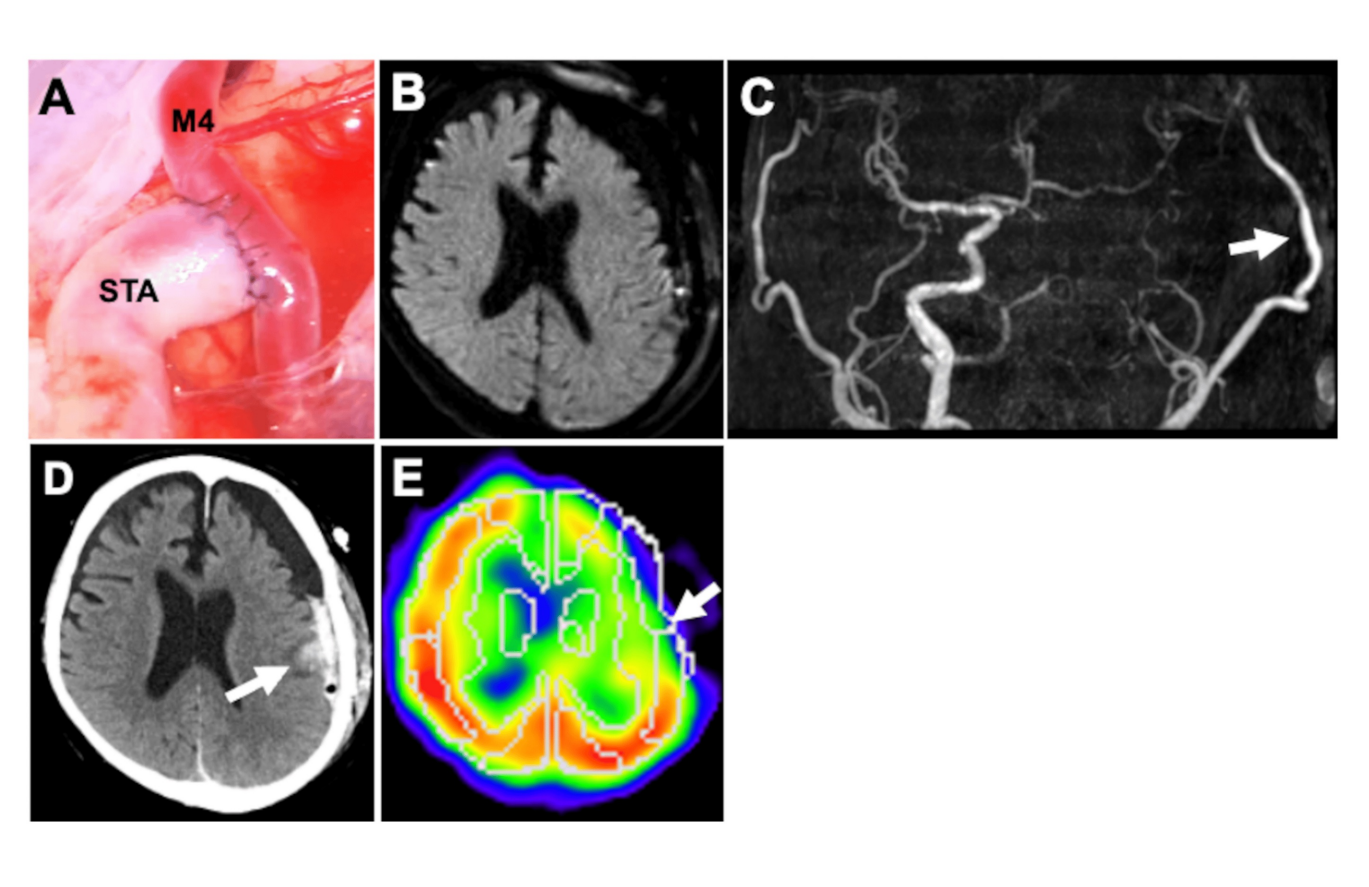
Donor-recipient caliber mismatch and hemorrhagic complication (A) Post-anastomosis, a significant caliber mismatch was observed between the donor STA and the recipient M4. (B) MRI and MRA on day 1 postoperatively revealed no stroke lesions in the brain parenchyma, (C) further demonstrating good bypass patency with strong signals of remarkably thick STA (white arrow). (D) CT on day 4 postoperatively revealed an ICH in the left temporal lobe (white arrow). (E) CBF near the site of anastomosis was not considered to fall within the range of hyperperfusion on ^123^I-IMP SPECT on postoperative day 5 (white arrow). STA: superficial temporal artery; MRI: magnetic resonance imaging; MRA: magnetic resonance angiography; ICH: intracerebral hemorrhage; CBF: cerebral blood flow; ^123^I-IMP: N-isopropyl-p-[^123^I]iodoamphetamine; SPECT: single-photon emission computed tomography
